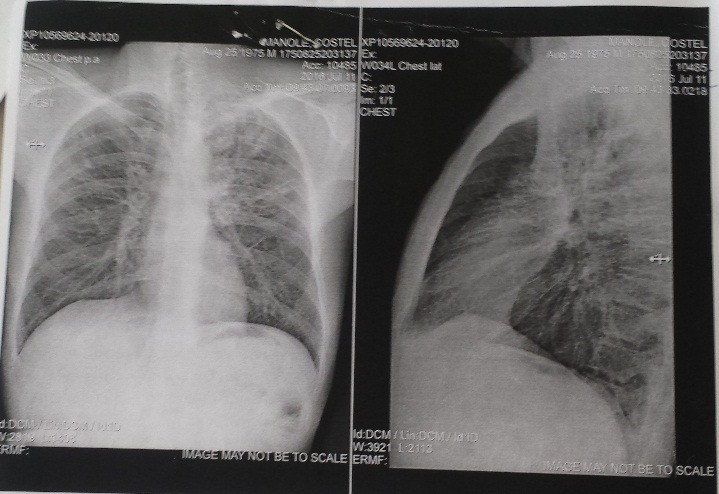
Thoracic X-ray: areas of non-homogenous condensation in both lungs, more prominent on the left side; bilateral hilar lymphadenopathy
